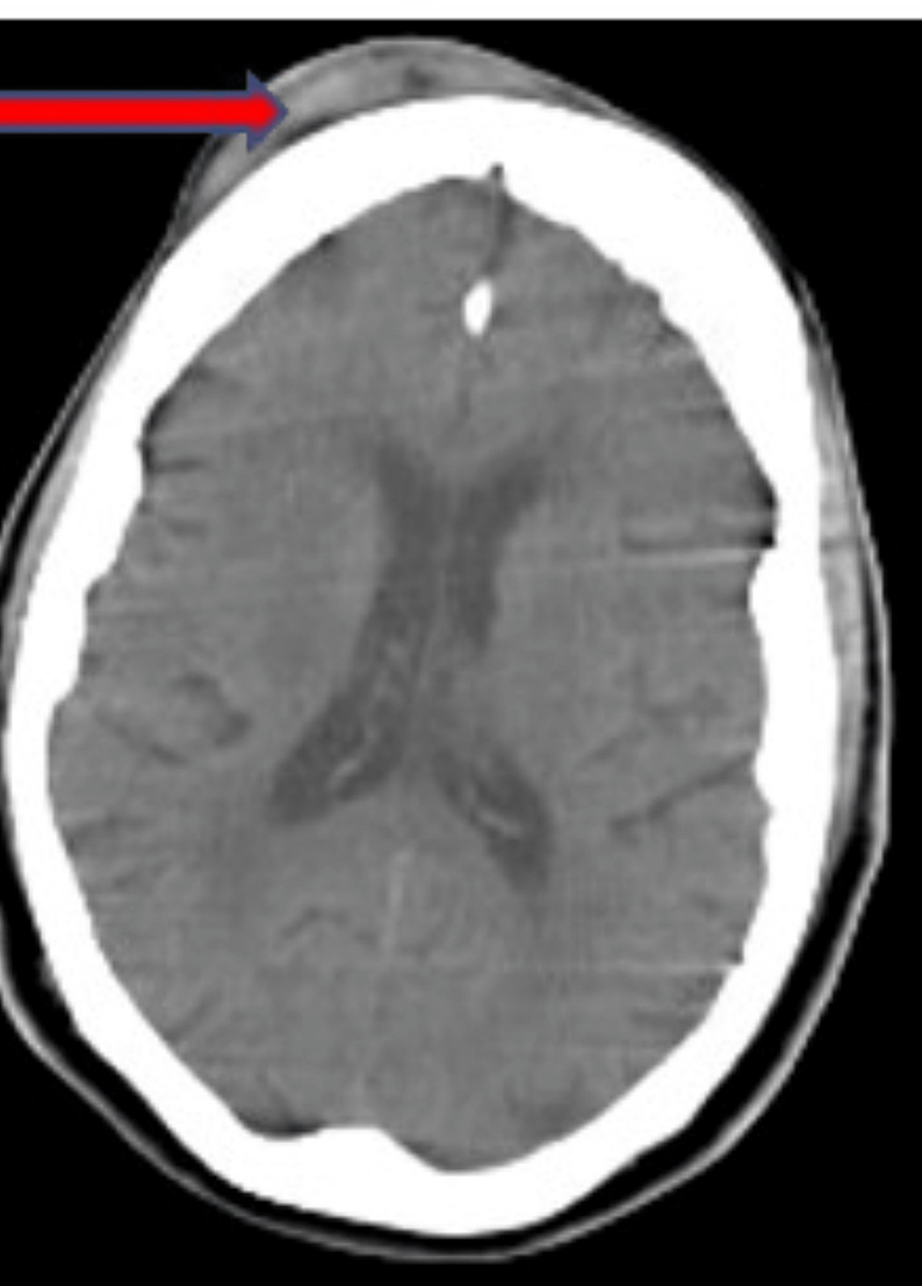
Head computed tomography scan without contrast showing a soft tissue hematoma in the right frontal location.
